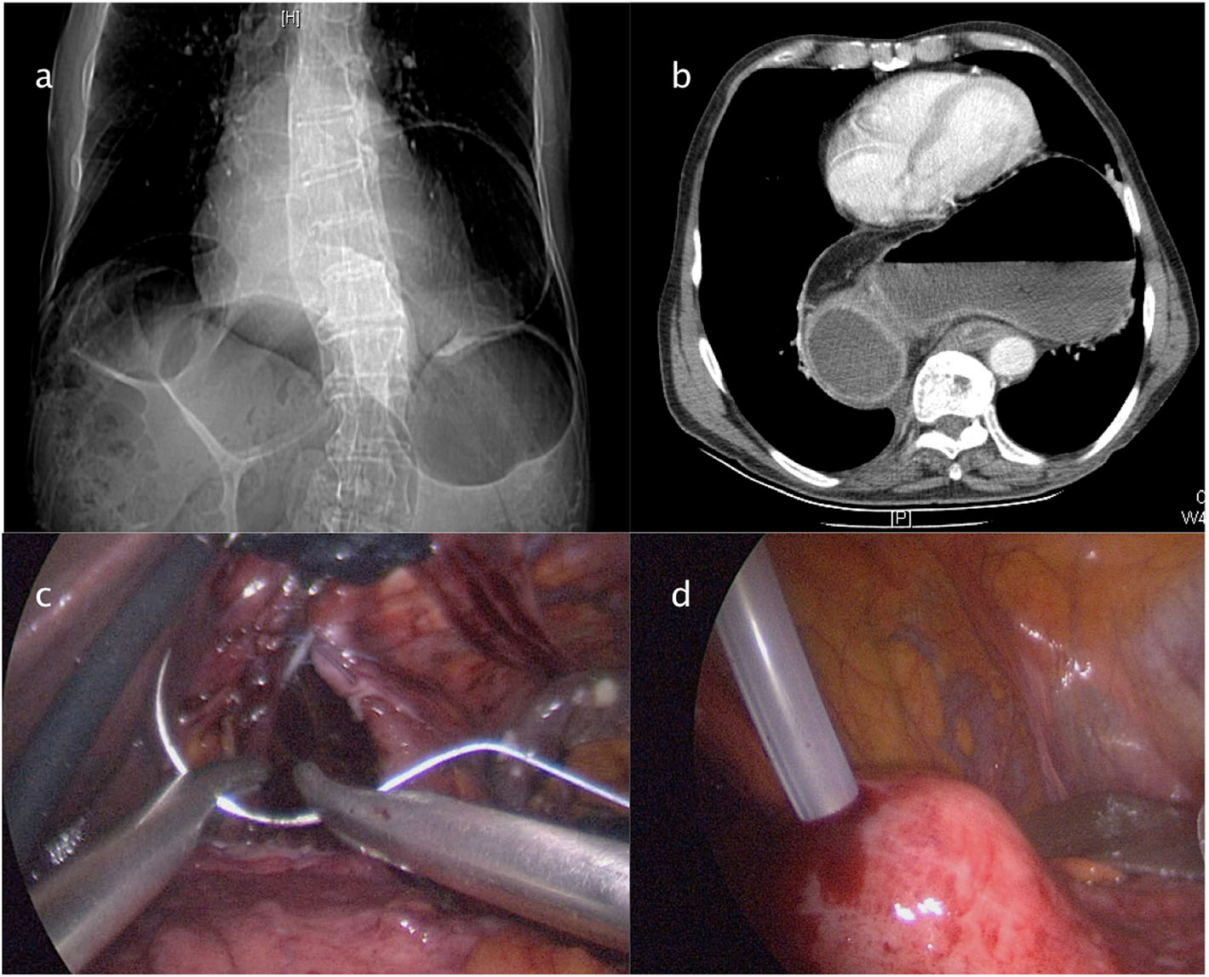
Case 3: Preoperative radiologic exams and endoscopic treatment. a Chest radiography showing migration of the stomach in the chest. b CT scan showing the stomach volvulus. c Crura closure by intracorporeal stitches. d Percutaneous endoscopic gastrostomy (PEG)
